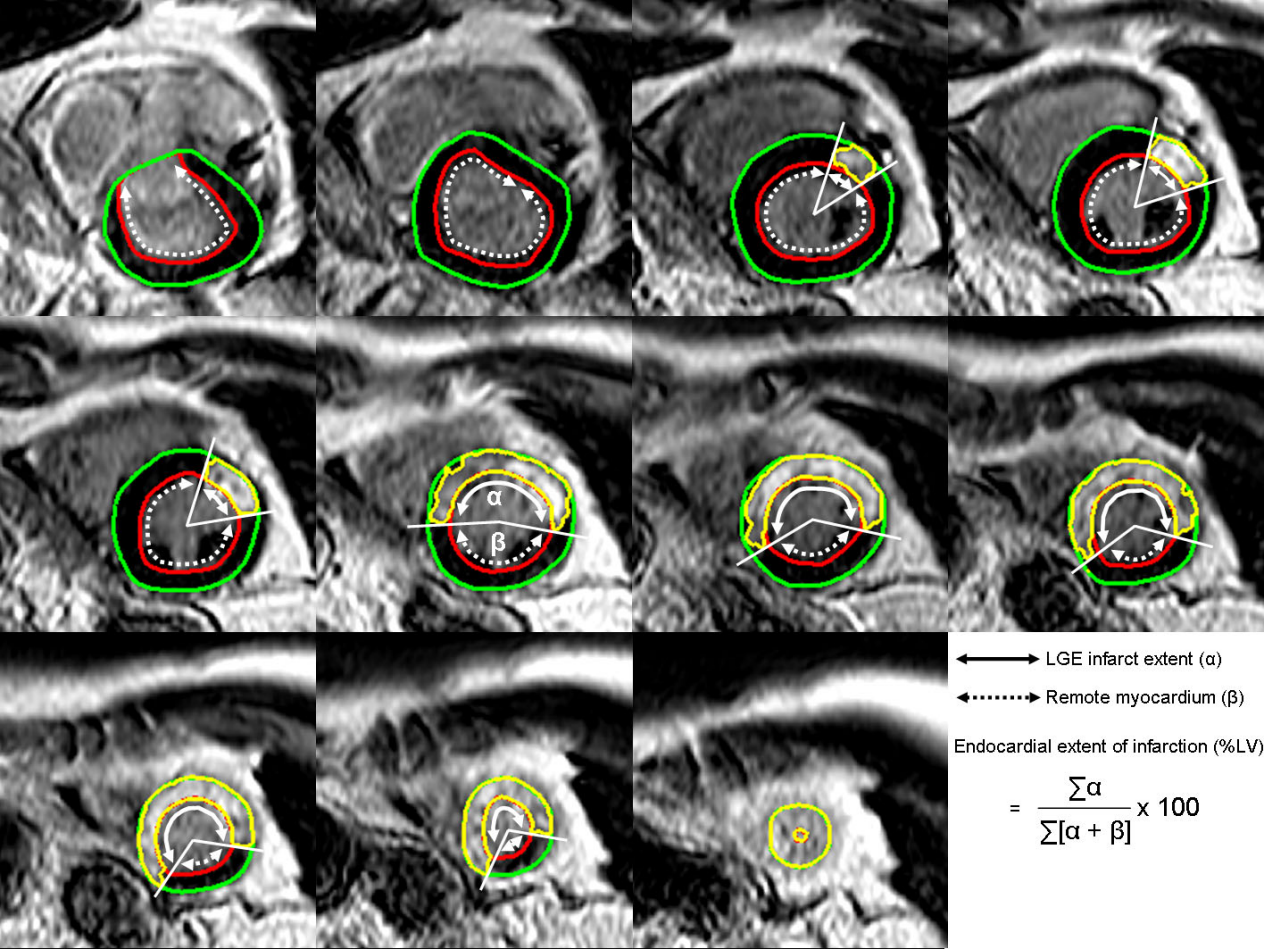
Endocardial extent of infarction. Late gadolinium enhanced (LGE) CMR showing short-axis slices covering base (top left) to apex (bottom right) in a patient with an occlusion of the left anterior descending coronary artery. The endocardial borders are traced in red and the epicardial borders are traced in green. The infarct is shown as an LGE area within the myocardial borders and is delineated in yellow. The circumferential endocardial extent of LGE is marked by the solid arrow (α) and the remote myocardium is marked by the dashed arrow (β). The endocardial extent of MI was defined as the sum of the LGE circumferential endocardial extent in each short-axis slice (∑α), divided by the total endocardial extent (∑ [α + β]) of the LV. In this patient, the MaR by T2-weighted imaging and LGE endocardial extent was 37% and 32%, respectively. The corresponding infarct size was 32%, resulting in a myocardial salvage of 13% and 1% when measured with T2-weighted imaging and LGE endocardial extent, respectively.
